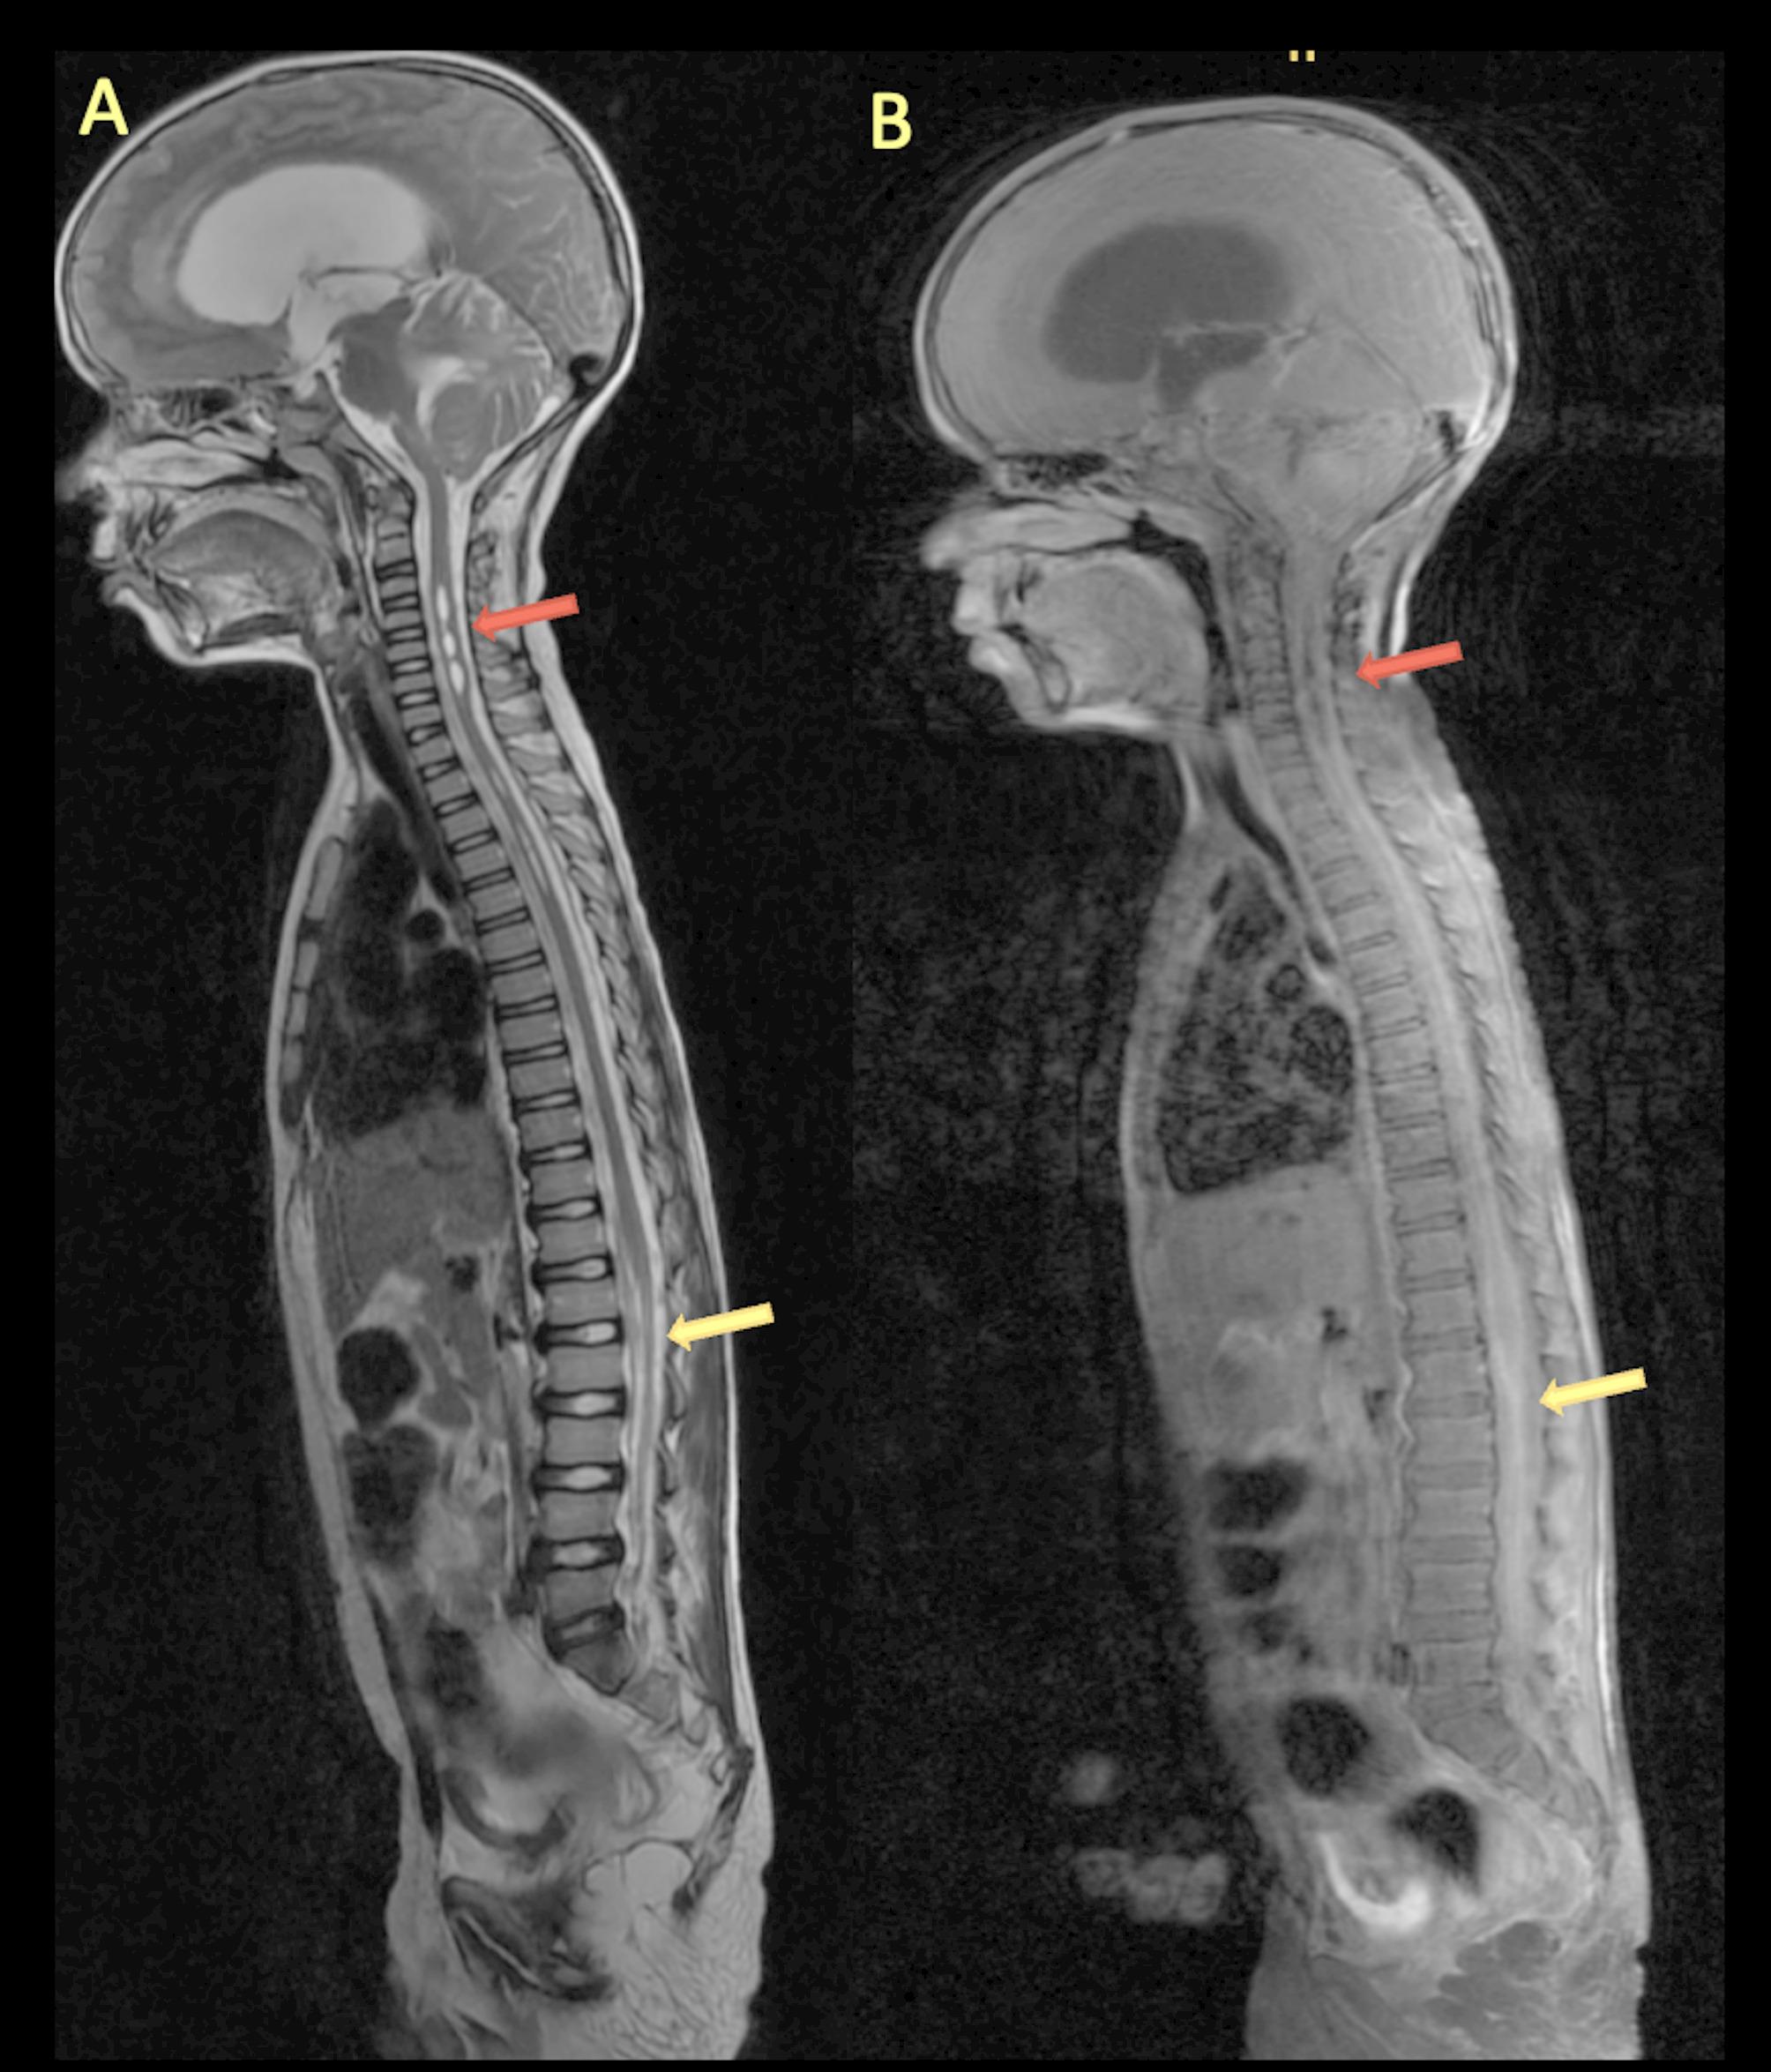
MRI spine. Sagittal T2 weighted image (A) showing dilatation of the central canal (hydrosyringomyelia) in cervical and thoracic cord (red arrow) and post contrast T1FS image (B) demonstrating extensive thickening and enhancement of the arachnoid, disappeared subarachnoid space, and nodular enhancement of conus medullaris (yellow arrow)
